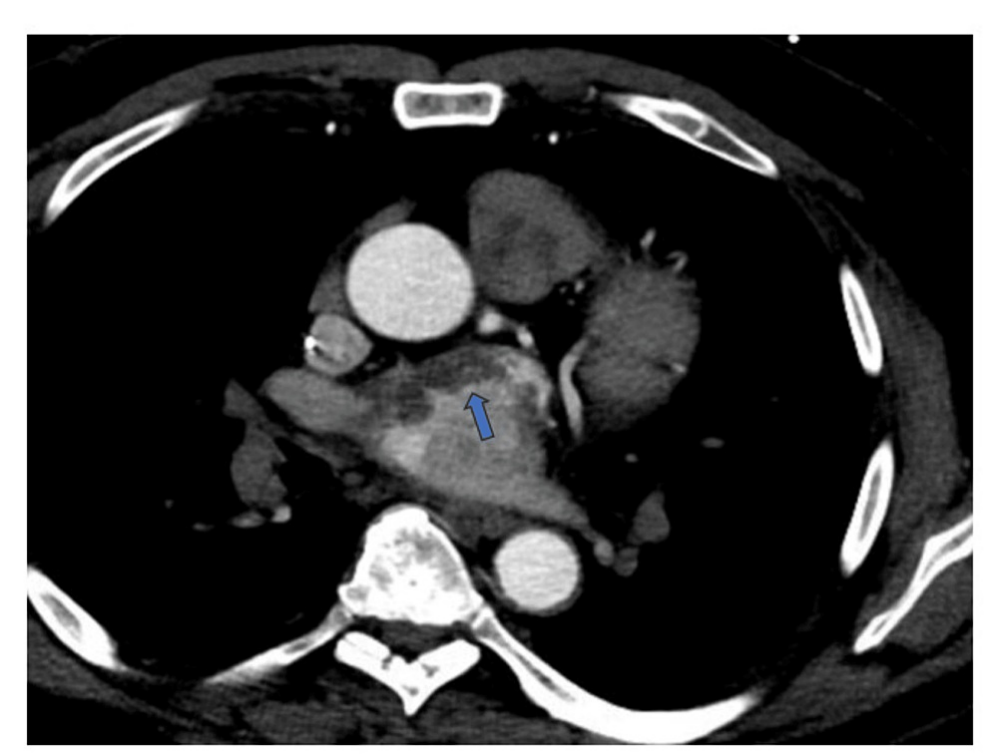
Computed tomography angiogram of the heart showing a left atrium mass
